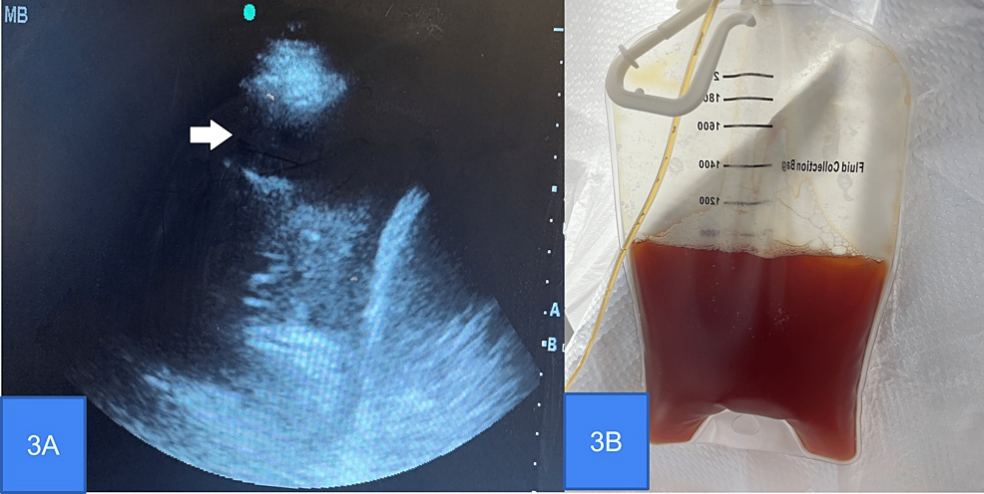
(A) Ultrasound image showing pleural effusion (white arrow); (B) Pleural drainage post thoracentesis showing serosanguineous pleural effusion.
